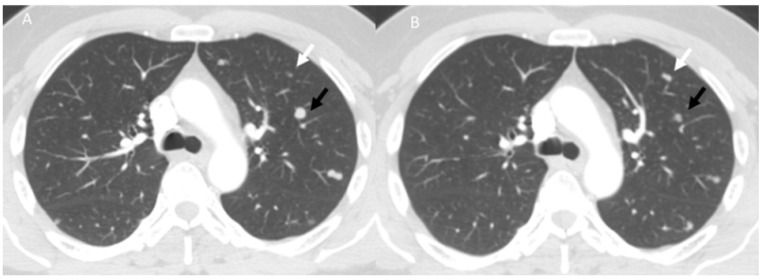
Dissociated responses in a melanoma patient during immunotherapy. CT assessment in pre-treatment phase (A) and at 3 months (B) follow-up: black arrows show the regression of the lesion while white arrows show the increase in lesion dimensions.
